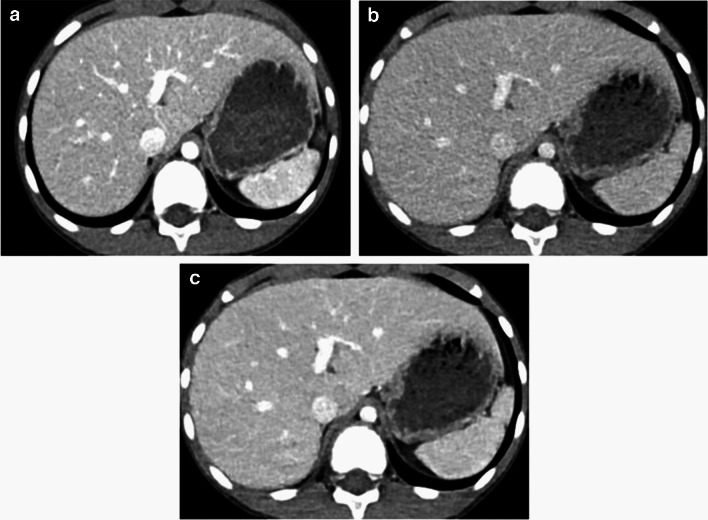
Axial contrast-enhanced computed tomography (CT) images of a 9-year-old boy with upper arm synovial sarcoma. Sequential abdominal CT was conducted within 2 months. a Standard-dose image shows excellent organ (liver, 167.6 ± 9.0 Hounsfield unit (HU)) and vessel (aorta, 327.0 ± 16.7 HU; portal vein, 324.8 ± 10 HU) enhancement with some grainy noise. b Low-dose image exhibits decreased organ (liver, 152.0 ± 12.1 HU) and vessel (aorta, 208.2 ± 12.6 HU; portal vein, 228.2 ± 14.9 HU) enhancement with persistent grainy noise. c Contrast-boosted image shows excellent organ (liver, 174.7 ± 9.5 HU) and vessel (aorta, 260.0 ± 11.9 HU; portal vein, 285.2 ± 12.1 HU) enhancement with reduced grainy noise. The qualitative organ enhancement scores for the standard-dose, low-dose, and contrast-boosted images were 4/4, 3/3, and 4/4, respectively (reader 1’s score/reader 2’s score). Vessel enhancement scores were 5/4, 3/3, and 4/4, whereas noise scores were 3/4, 3/3, and 4/4. Overall image quality scores were 4/4, 4/3, and 4/4
